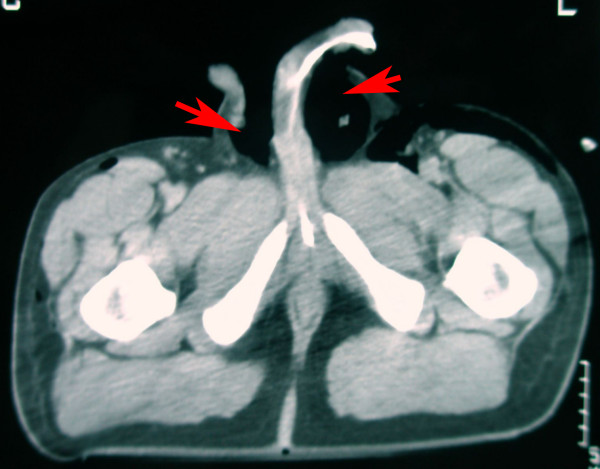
CT demonstrates pneumoscrotum (red arrows).
